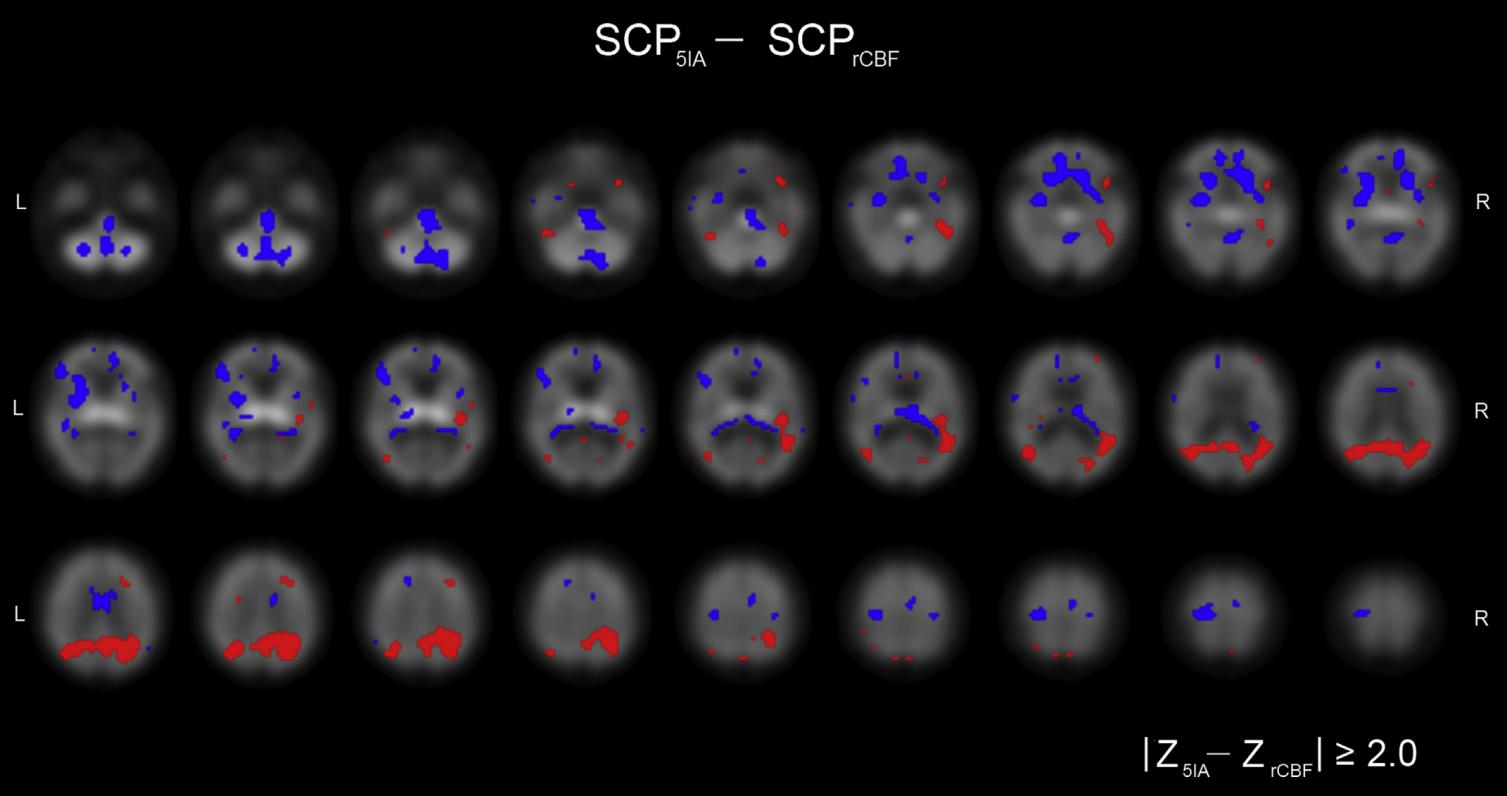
Difference image between the nicotinic and regional cerebral blood flow spatial covariance patterns displayed axially and superimposed upon the ^123^I-5IA-85380 SPECT template. Red and blue regions represent (Z_5IA_ − Z_rCBF_) ≥ 2.0 and (Z_5IA_ − Z_rCBF_) ≤ −2.0, respectively. Abbreviations: L, left; R, right; rCBF, regional cerebral blood flow; SCP, spatial covariance pattern. (For interpretation of the references to color in this figure legend, the reader is referred to the Web version of this article.)
